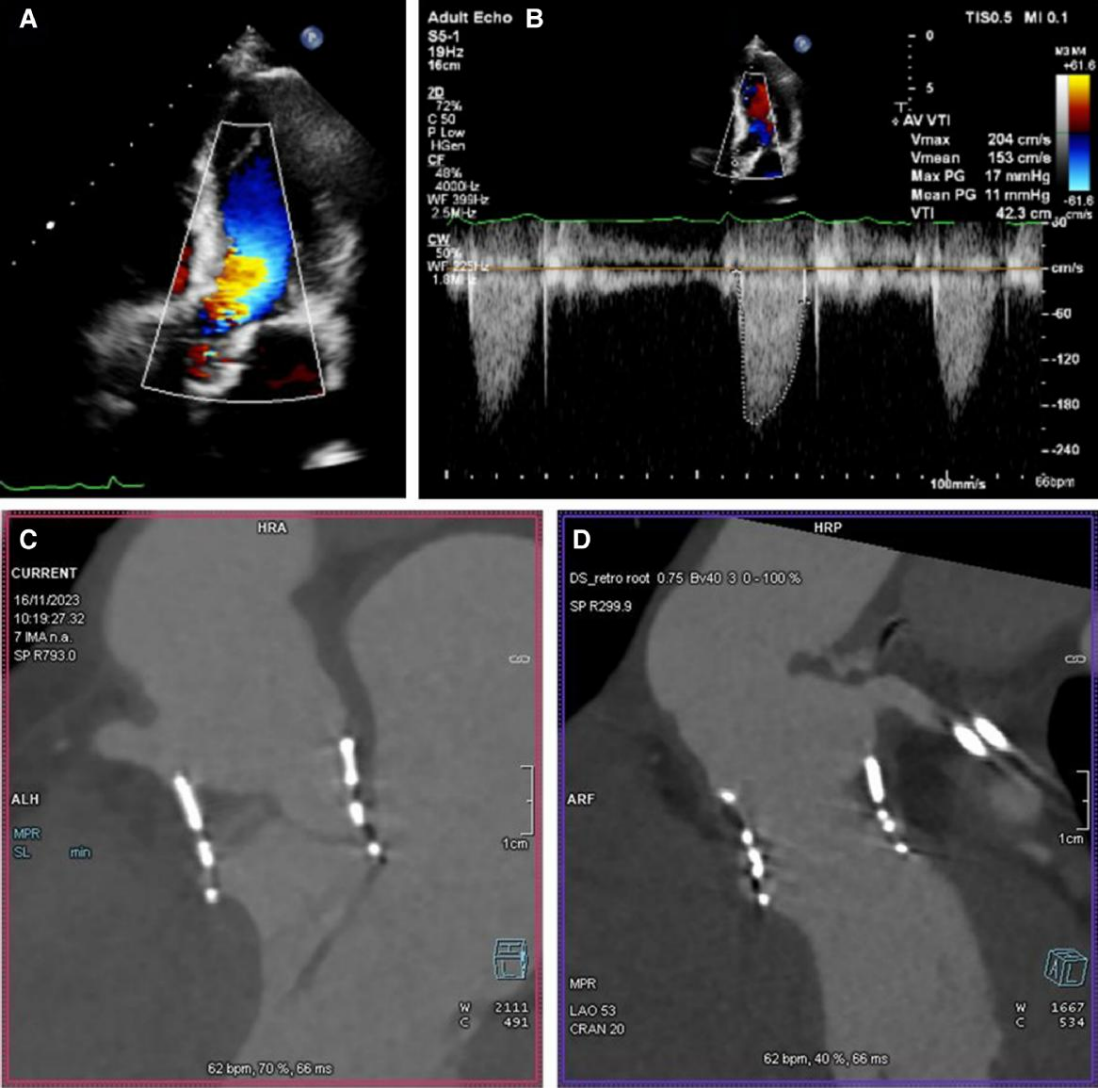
Transthoracic echocardiography 4 months post-transcatheter heart valve (THV) implantation: no residual aortic regurgitation by colour flow Doppler (*A*) and satisfactory transvalvular gradients (*B*); (*C* and *D*) CT scan demonstrating no migration of the THV and preservation of coronary access (left main coronary artery panel *C*, right coronary artery panel *D*).
